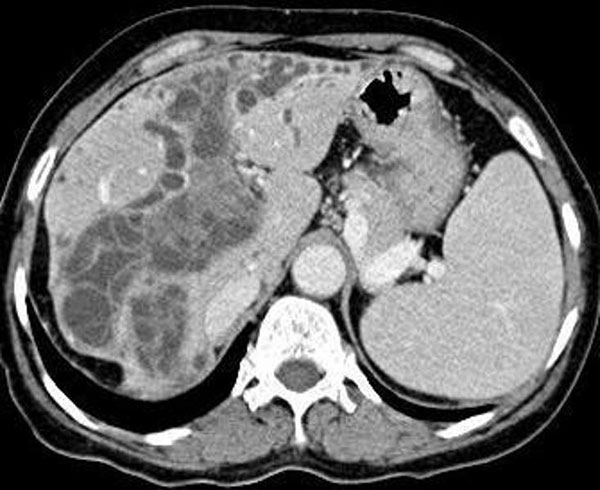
CT image showing dilated bile ducts which contain lineer hyperdens material corresponding to germinative membranes and other cyst contents.
